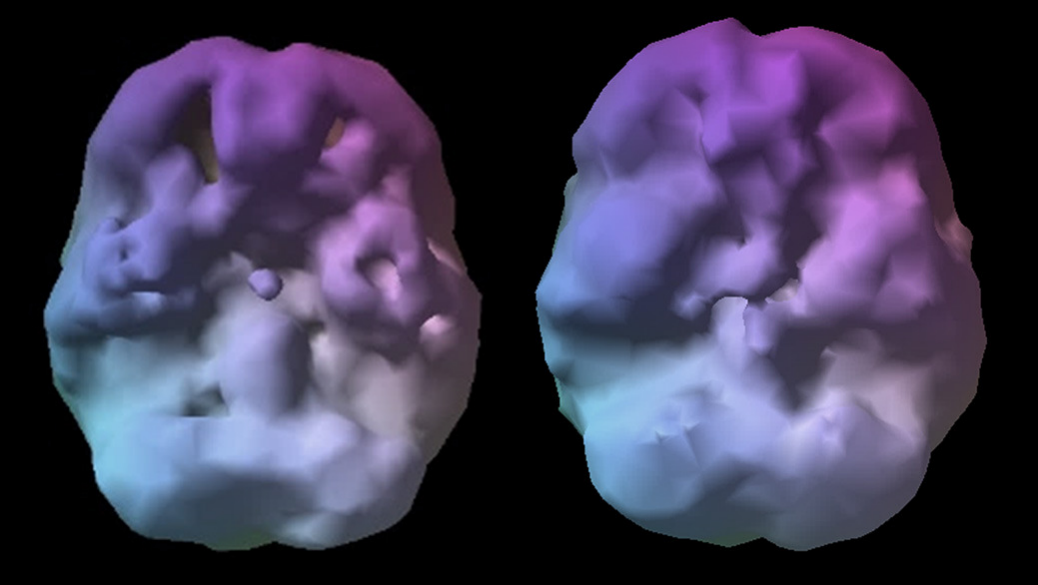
C's SPECT surface underside view images before (L) and after (R) treatment. C's SPECT image before treatment (L) reveals low activity, especially in the prefrontal cortex and temporal lobes compared to post-treatment image (R) showing overall improvement.
